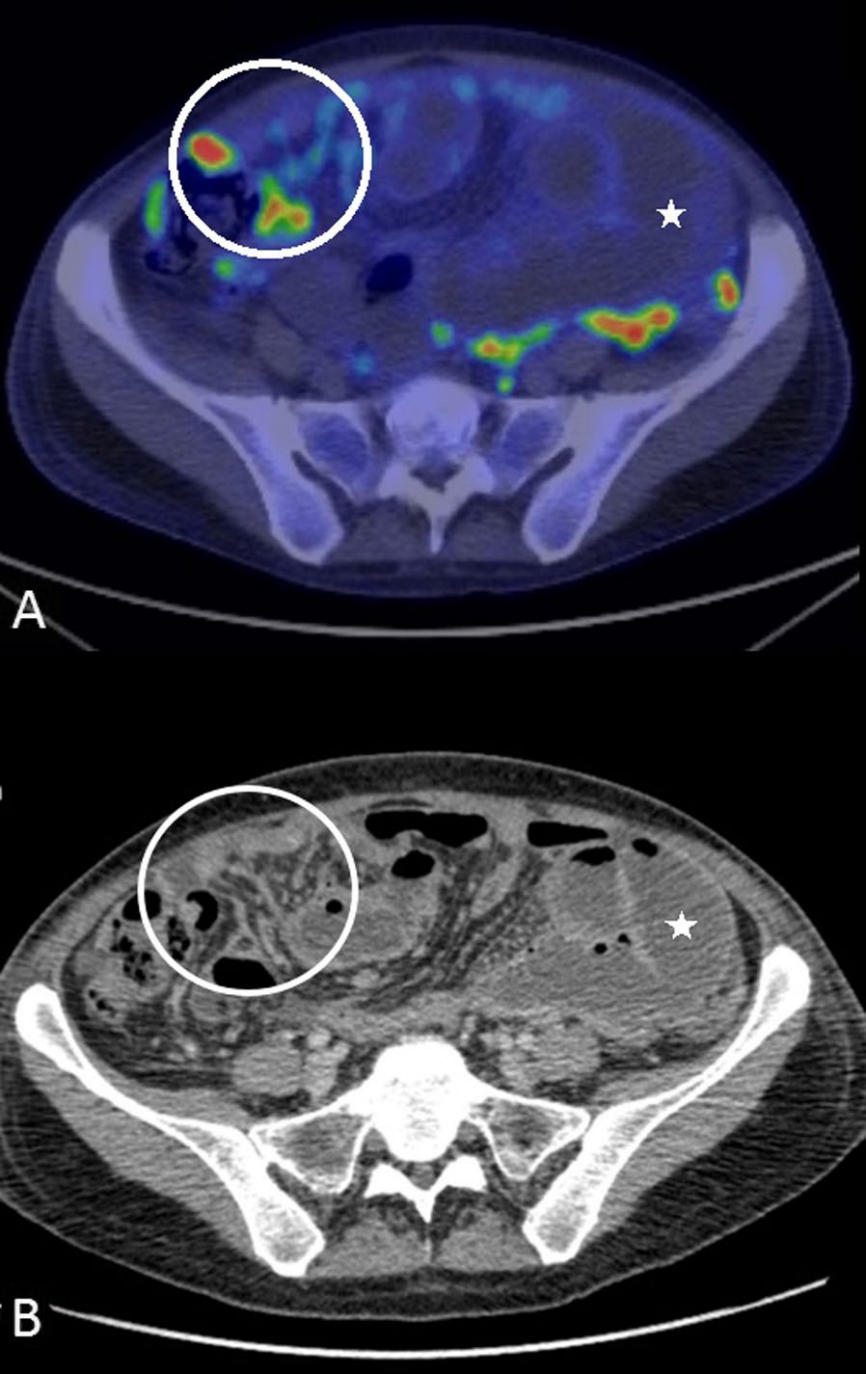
a, b: a PET-CT and (b) CT images of a patient with epithelioid malignant peritoneal mesothelioma showing nodular mesenteric fold thickening (white circle) and segmental small bowel obstruction(*)
